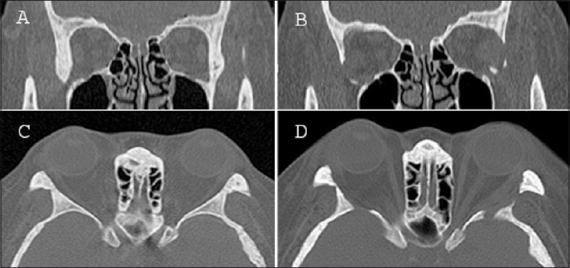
(A,B) Coronal CT scan preoperativel and 2 months postoperatively. (C,D) Axial scan for pre and postoperative orbit, showing more lateral wall decompression in the left side
